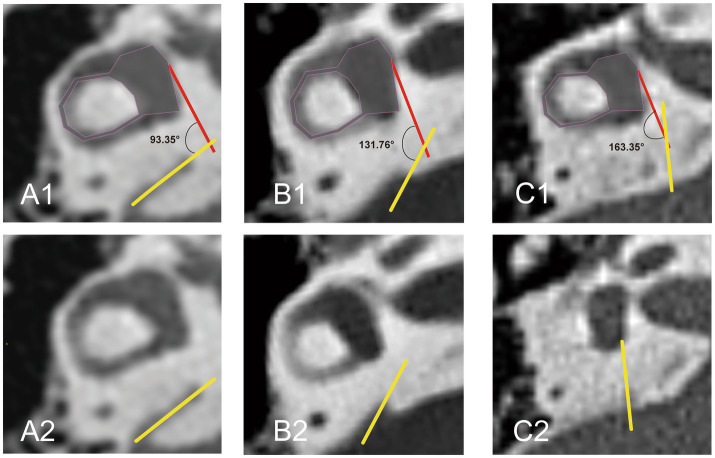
The measurement of the angular trajectory of the vestibular aqueduct (ATVA). The ATVA measurement of a 37-year-old male ipsilateral delayed endolymphatic hydrops patient (A1,A2), a 36-year-old female control participant (B1,B2), and a 52-year-old male Ménière’s disease patient (C1,C2) using two specific CT images, respectively. The angle between the red line and yellow line is the ATVA.
